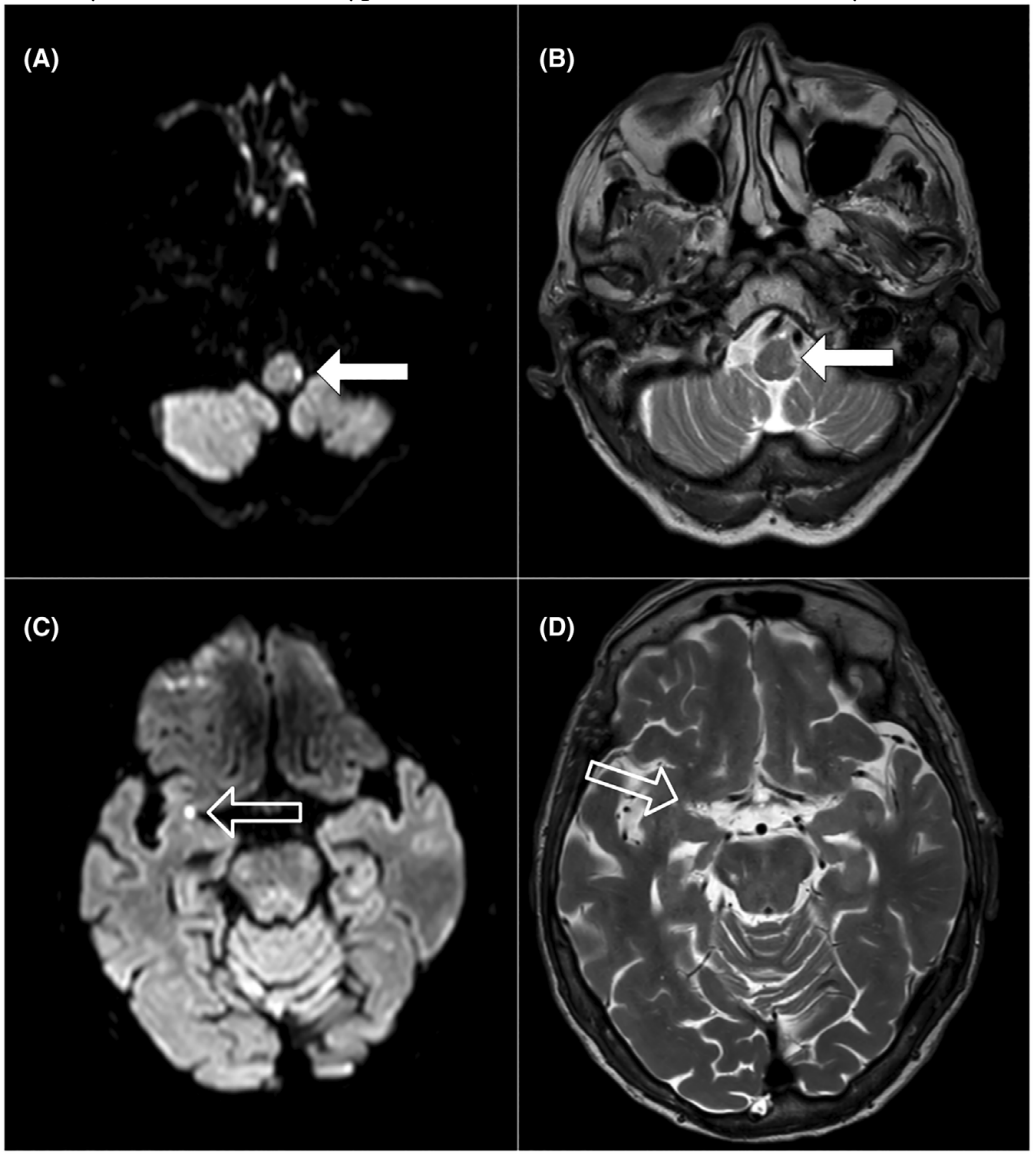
MRI (3T, Ingenia CX, Philips) acquired 14 days after symptom onset demonstrates acute ischemic lesions with diffusion‐weighted imaging (DWI) restriction and T2‐weighted (T2W) hyperintensity in the left medullary region (white arrows A and B) and in the right subinsular region (translucent arrows C and D).
